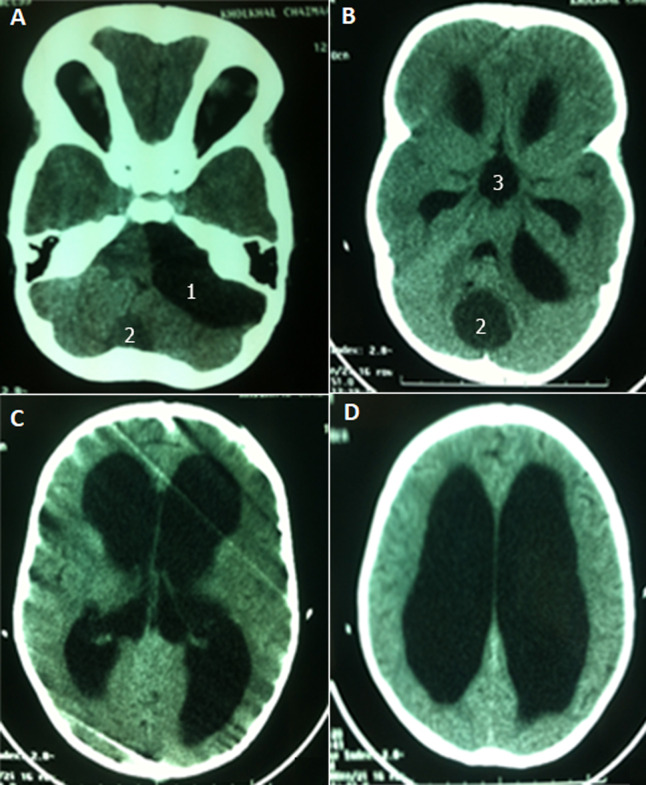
(A,B,C,D) axial non contrast CT scan showing CPA arachnoid cyst of the cerebellopontine angle (1), a vermian dermoid cyst (2) and significant dilatation of the third ventricle and lateral ventricles
